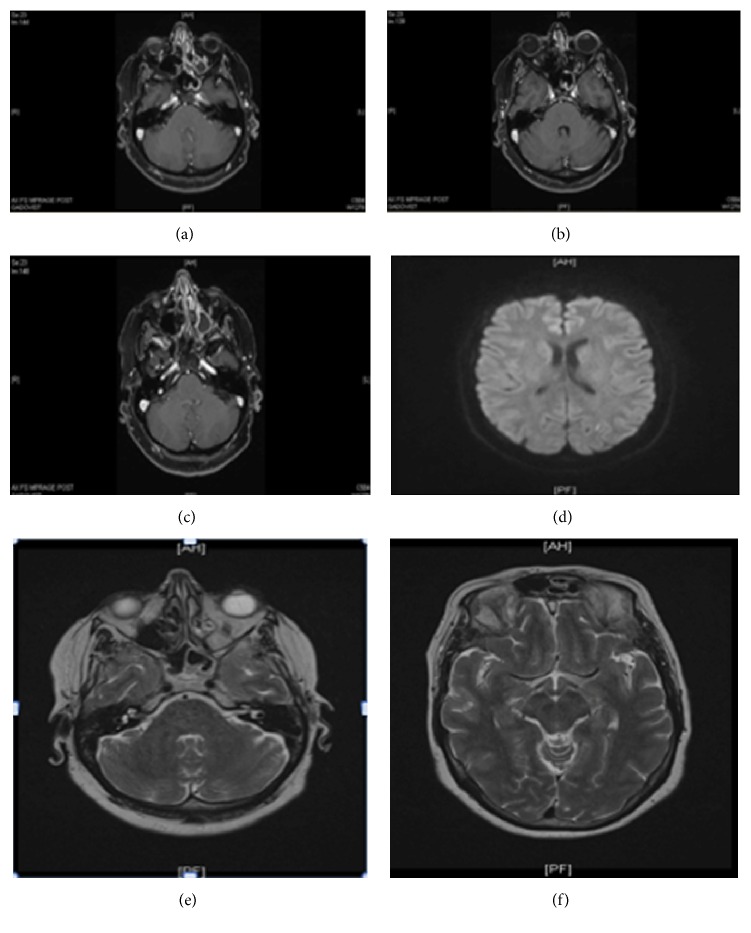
Brain magnetic resonance imaging (MRI) with contrast 6 weeks later. (a, b) T1 (magnetization-prepared rapid acquisition with gradient echo): tiny focus of enhancement seen within the superior portions of the right internal auditory canal. (c) T1 (magnetization-prepared rapid acquisition with gradient echo): tiny focus of enhancement seen at the posterior portions of the basal turn of the cochlea. (d) Diffusion Weighted Image: left superior occipital white matter with restricted diffusion. (e) T2-weighted sequence: no enhancement at the cerebellum and pons. (f) T2-weighted sequence: no enhancement at the midbrain.
